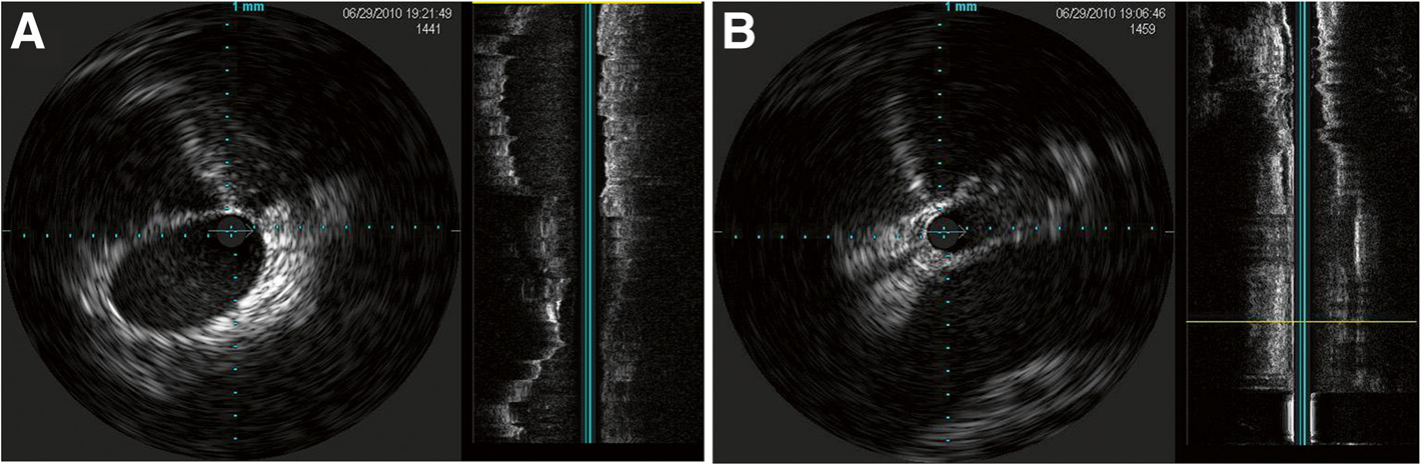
Example of intravascular ultrasound in the internal jugular vein. Normal patent lumen (A) and stenotic lumen (B).
